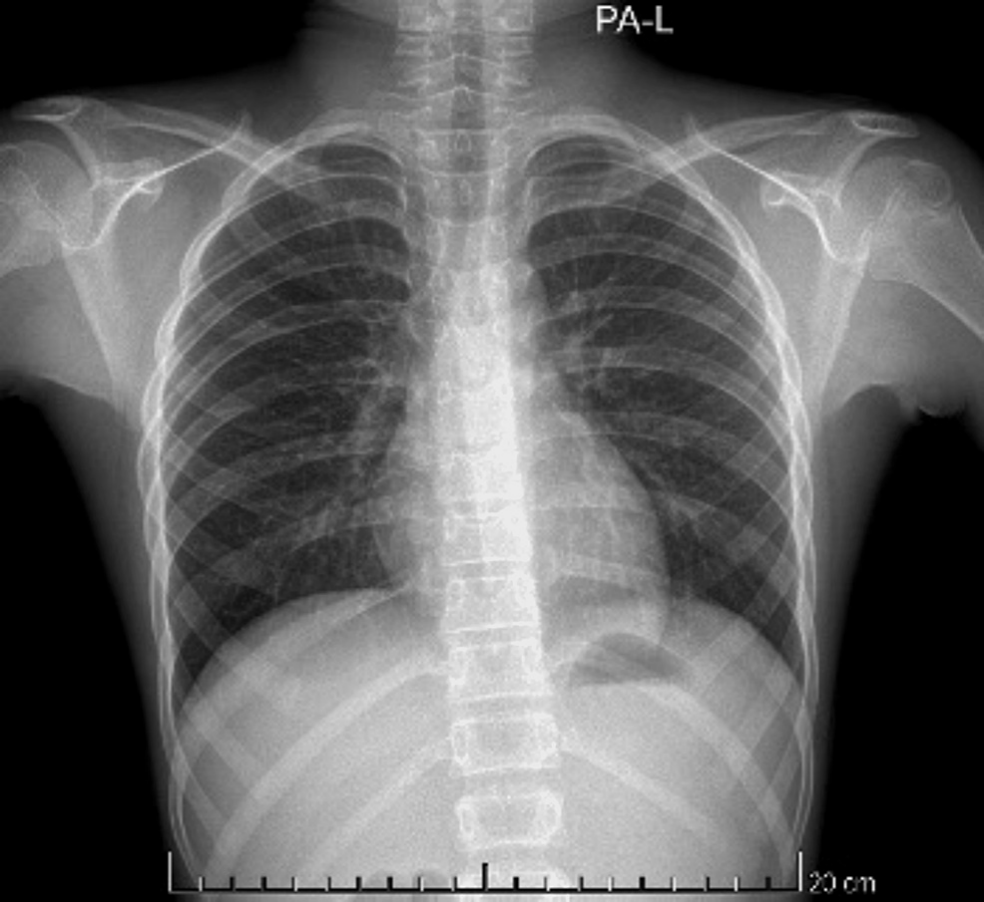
Follow up chest radiograph Radiograph taken two weeks after discharge shows complete resolution of previously noted air collections
